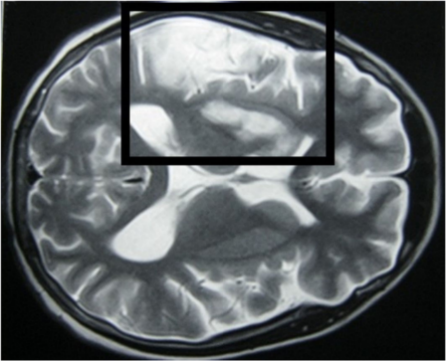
Magnetic resonance image: transverse section of the brain 1 month after the onset of illness. This section shows new involvement of the right cerebral cortex (right middle meningeal arterial territory) with vasogenic edema
